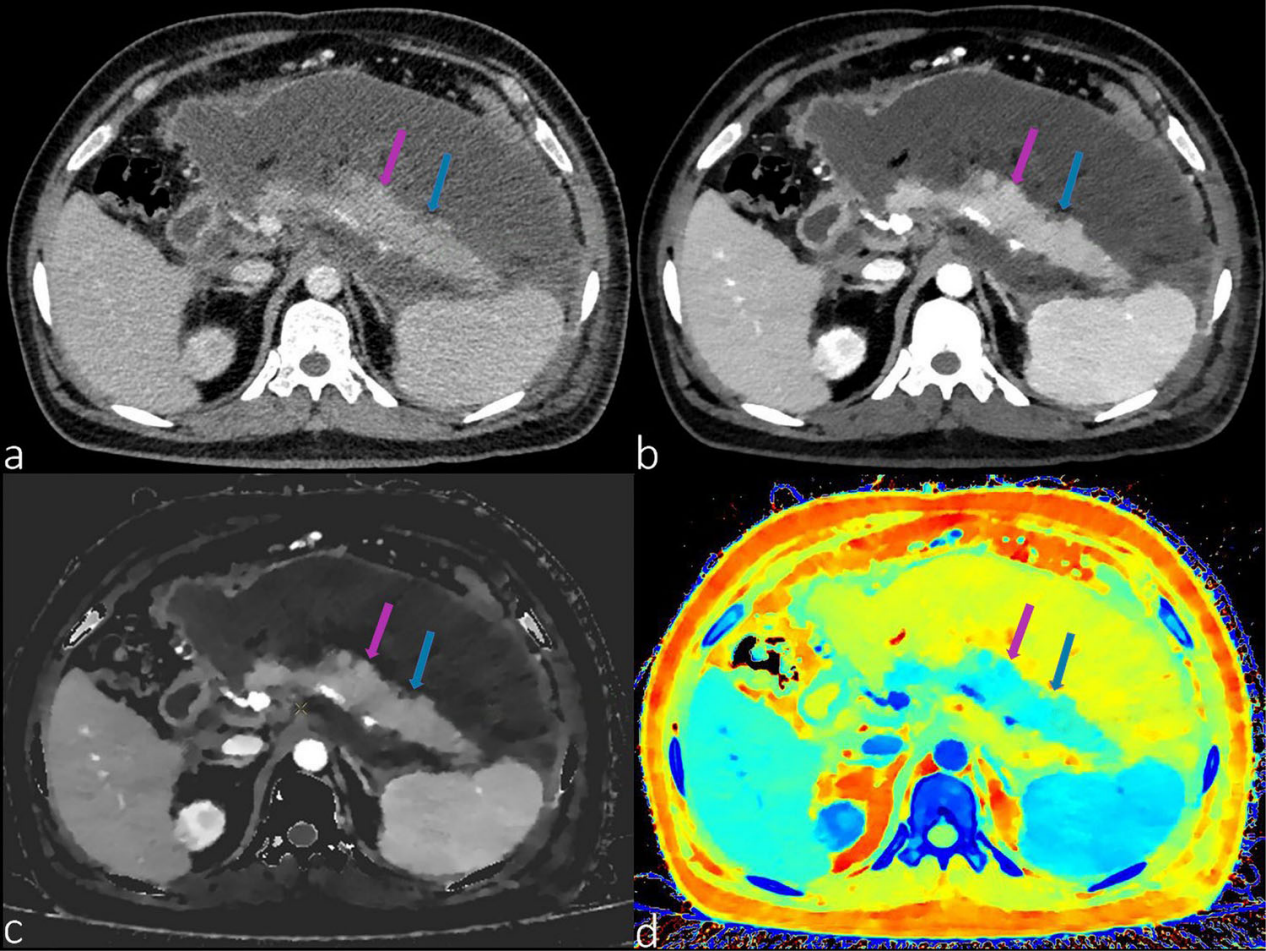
A 36-year-old male patient presented with abdominal pain for 12 days, aggravated with dyspnea for 8 days. He was diagnosed with necrotic pancreatitis. Portal phase CT and postprocessing images: a conventional axial images; b 40 keV images; c
